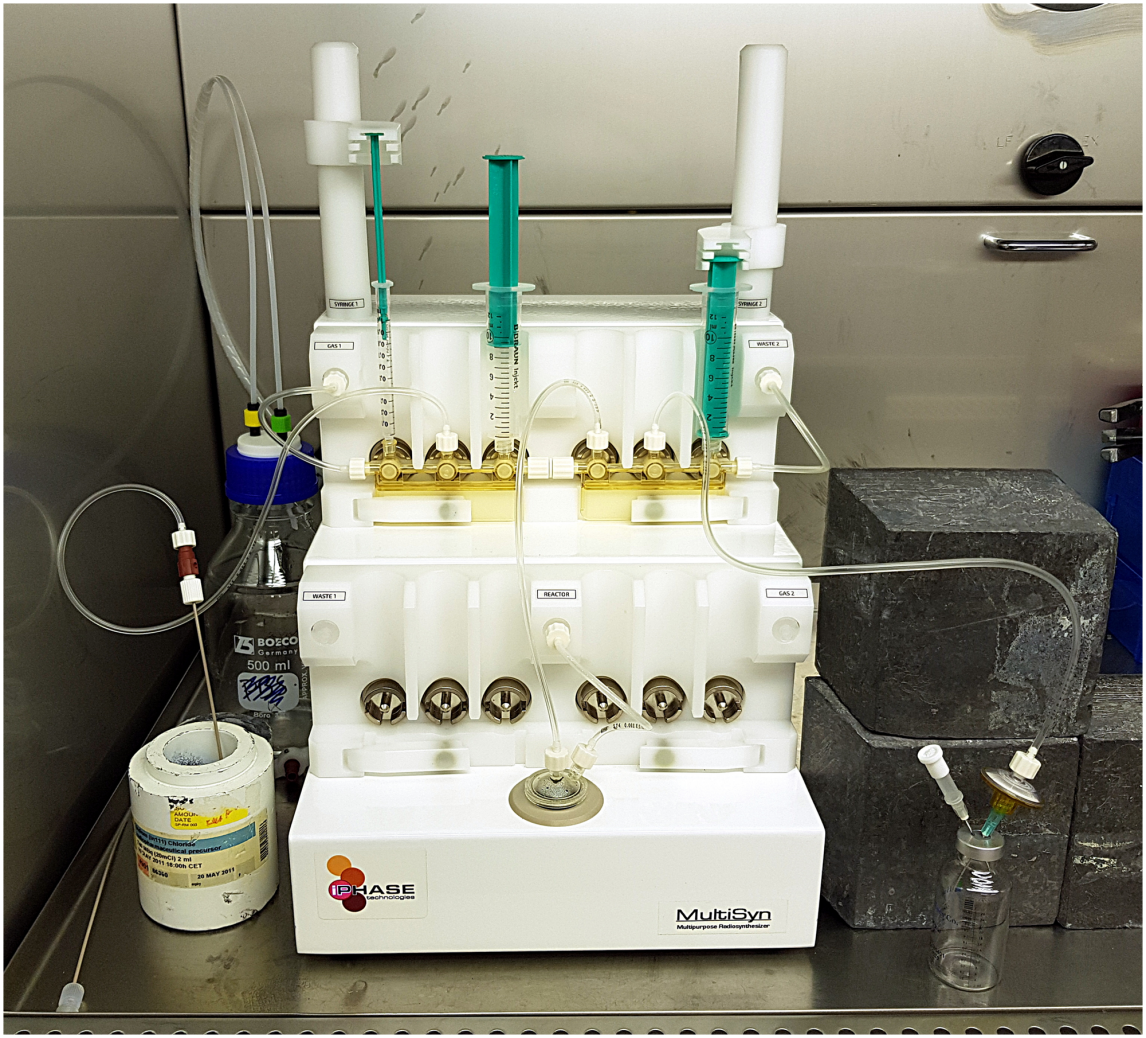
Picture of iPHASE MultiSyn set‐up for automated radiosynthesis of [^177^Lu]Lu‐PSMA‐617 according to Figure 4 and Table 1
